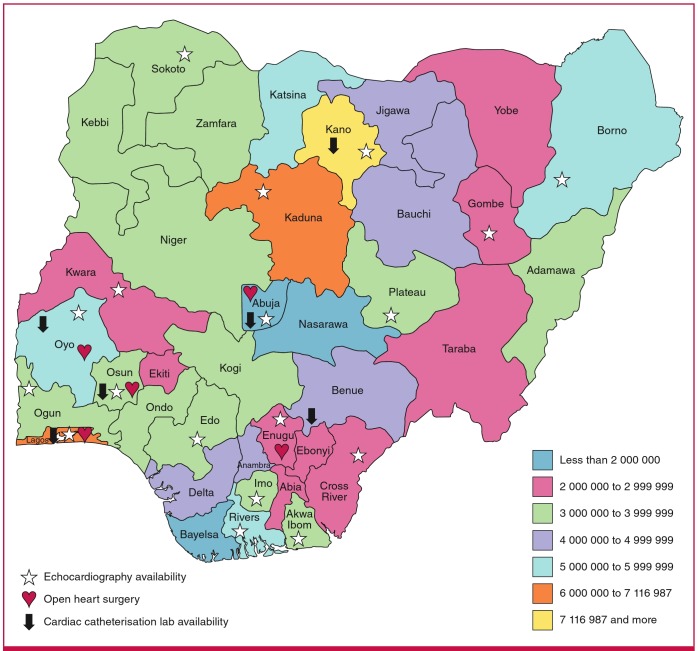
Population map of states with echocardiography, cardiac catheterisation and open-heart surgery facilities.
